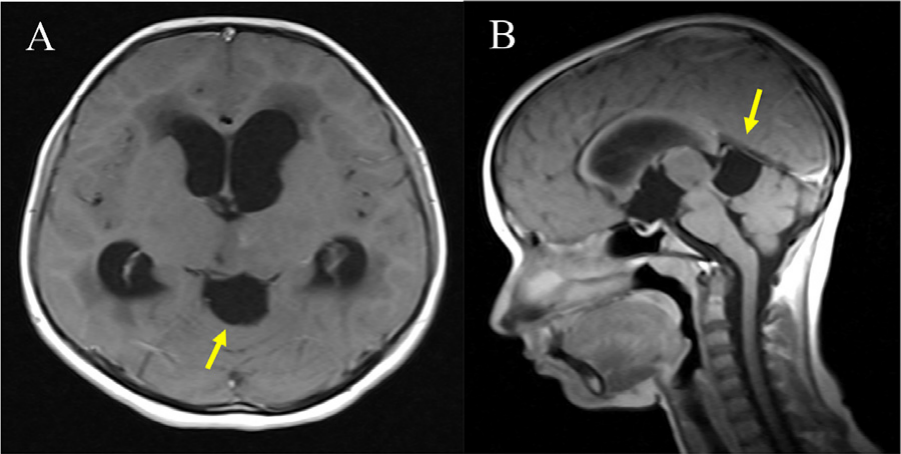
Axial (A) and Sagittal T1 (B) post contrast MRI showing no enhancement of cyst walls and no mural nodule on post Gadolinium administration.
